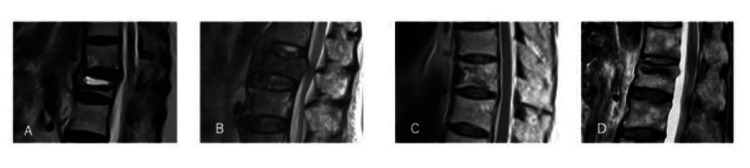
Four types of MRI T2WI classification (A) Locally confined high intensity; (B) Diffuse low intensity; (C) Mosaic; (D) Other lesions.
